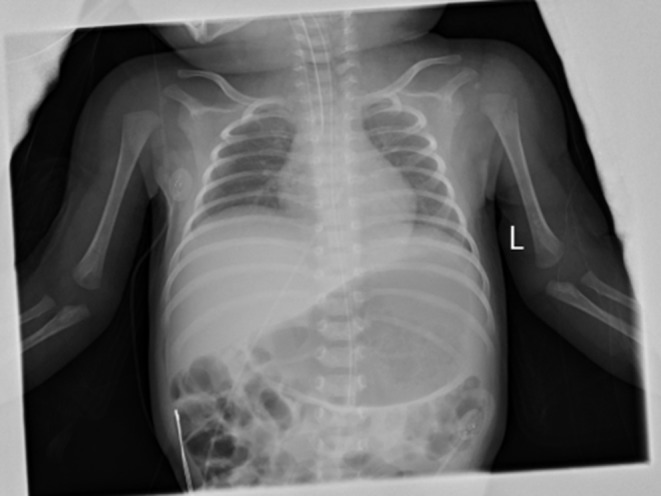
Anterio-posterial Chest and abdominal X-ray on DOL 23. The anterio-posterial chest and abdominal X-ray on DOL23 showed a slightly decreased lung volume with normal lung parenchyma and heart image. The right diaphragm was at the right 6–7 intercostal space and was 1 intercostal space higher than the left side.
